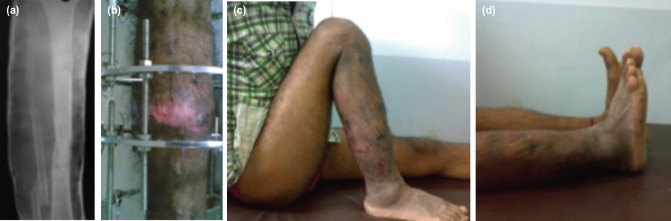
(a) Final follow-up radiograph of a case treated by IBT. (b) clinical picture before ring removal. (c) Clinical picture showing ROM of knee flexion. (d) clinical picture showing ROM of ankle plantar flexion. (e) Clinical picture showing ROM of ankle dorsiflexion.
